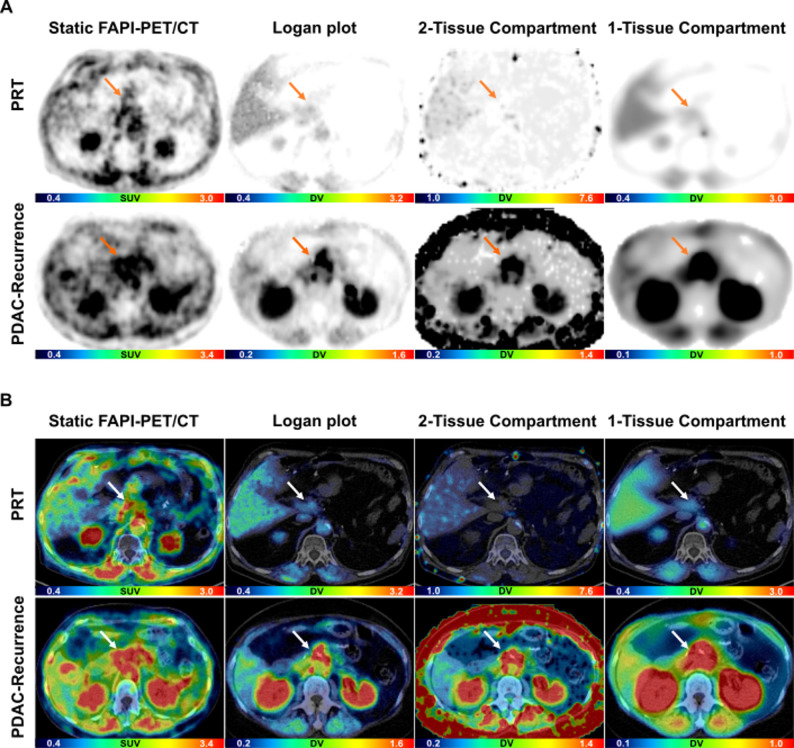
Representative static and parametric imaging data with ^68^Ga-FAPI-46-PET/CT of one patient with PR and one patient with PDAC-Recurrence. A PET imaging data with ^68^Gallium- (^68^Ga) labeled fibroblast activation protein inhibitor-46 (FAPI-46) of a patient with post-pancreatic surgery reactive tissue (PRT) and patient with recurrence of a pancreatic ductal adenocarcinoma (PDAC). B Corresponding fused PET/CT imaging data. SUV = standardized uptake value (unit less), DV = distribution volume (ml/cm^3^). Arrows indicate PRT and PDAC-Recurrence, respectively
